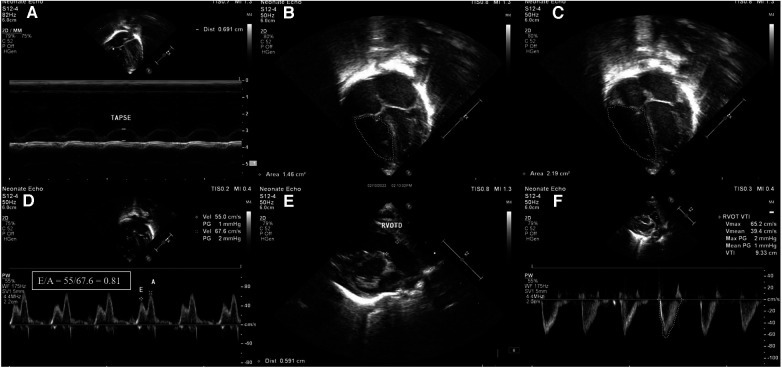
Images of right ventricular systolic and diastolic function parameters in echocardiography. (A) Tricuspid annular plane systolic excursion (TAPSE) shown in M-mode. (B) Fractional area change (FAC) in apical 4 chamber view-end systolic area (1.46 cm^2^). (C) Fractional area change (FAC) in apical 4 chamber view-end diastolic area (2.19 cm^2^). (D) Apical 4 chamber view pulse wave Doppler across tricuspid valve showing E/A ratio of 0.81. (E) Measurement of RVOT diameter (RVOTD) in parasternal long axis view (PLAX). (F) Right ventricular velocity time integral measured in short-axis view using pulse wave Doppler (VTI). Stroke volume = cross-sectional area × velocity time integral.
